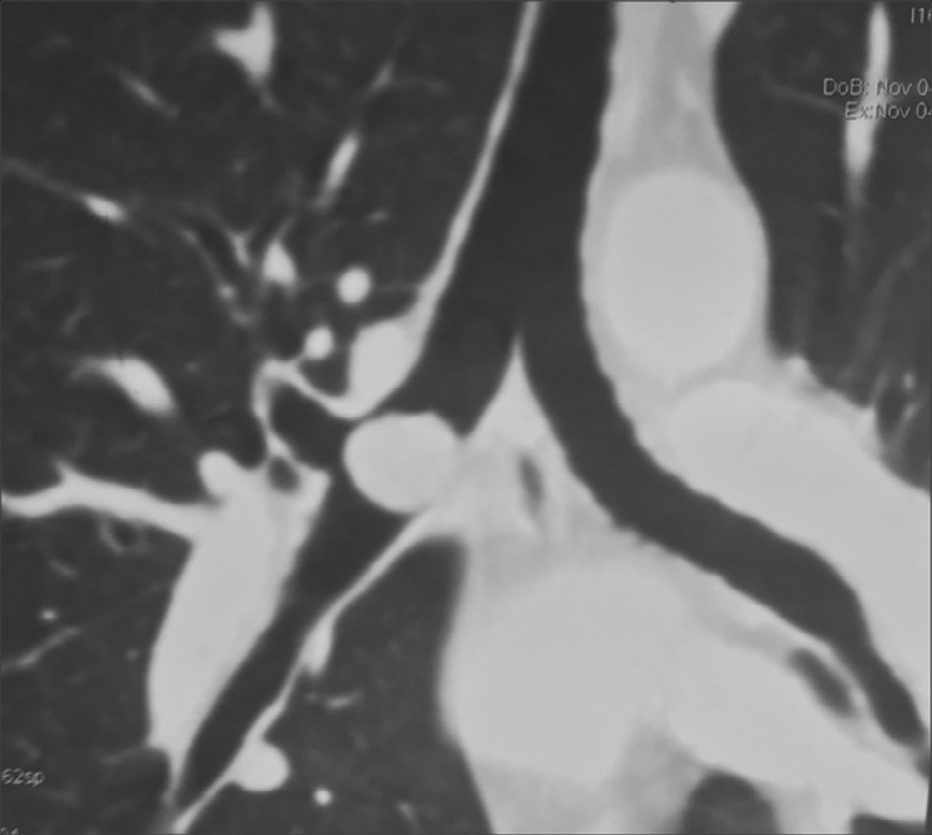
Computed tomography scan showed well‐circumscribed endoluminal formation in the posterior wall of primary right bronchus measuring 2 cm.
